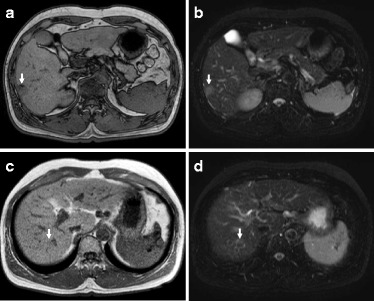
Abdominal magnetic resonance imaging (MRI) of the two tumors. a The tumor in the 6^th^ segment of the liver showed low intensity on T1-weighted images (*arrow*). b The tumor in the 6^th^ segment of the liver showed high intensity on T2-weighted images (*arrow*). c The tumor in the 7^th^ segment of the liver showed low intensity on T1-weighted images (*arrow*). d The tumor in the 7^th^ segment of the liver showed high intensity on T2-weighted images (*arrow*)
